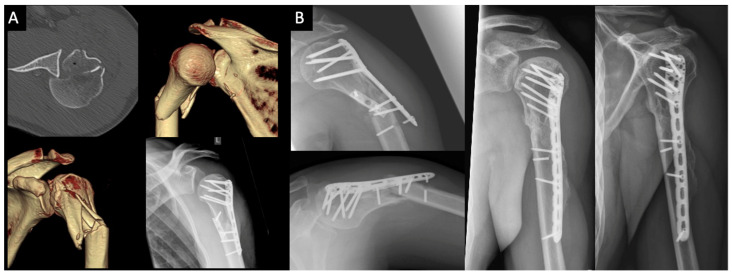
39-year old male bicyclist that collided with a motor vehicle; (A) axial CT scan and 3D reconstruction of a posteriorly dislocated surgical neck fracture with an additional reverse Hill-Sachs lesion; (B) secondary dislocation with screw failure (left) and final result after surgical revision 5.5 years after the index injury (right).
